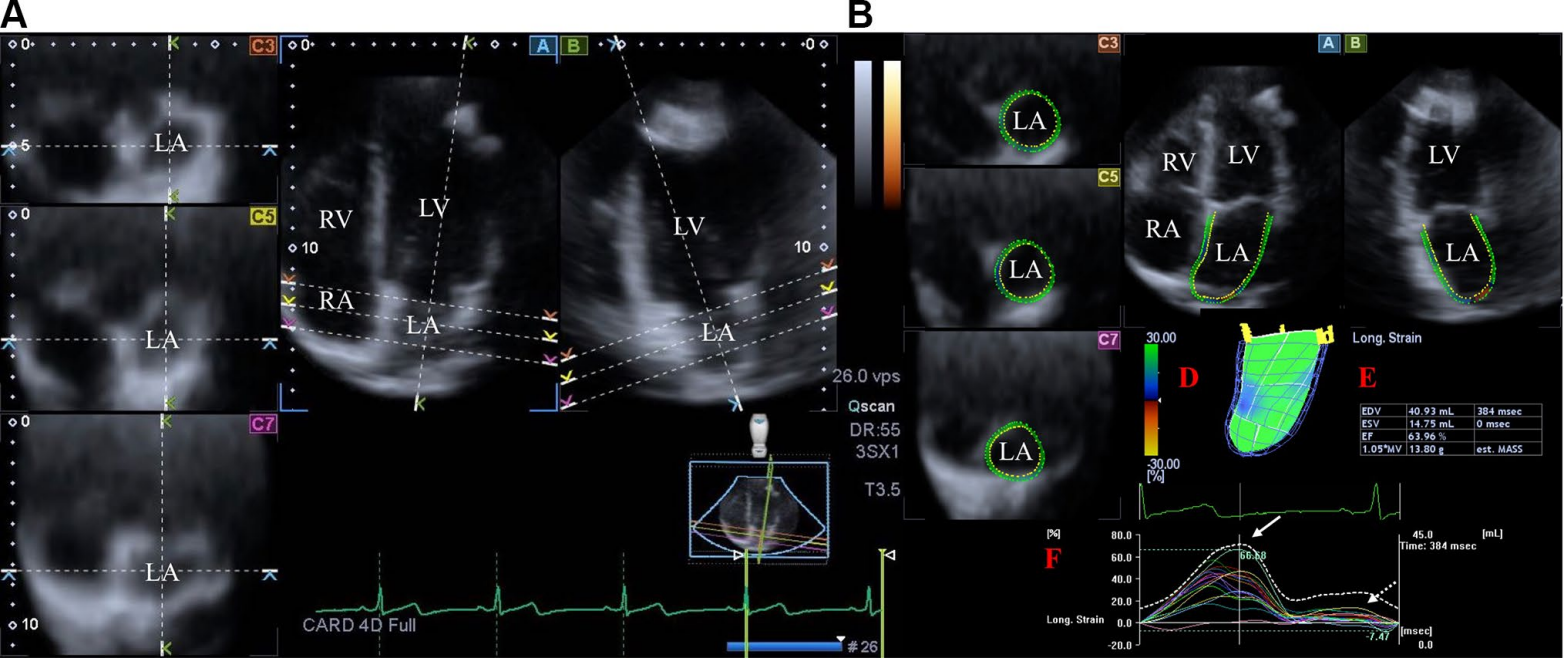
Images from three-dimensional (3D) full-volume dataset showing the left atrium (LA) in a healthy subject are demonstrated (a, b): (*A*) apical four-chamber view, (*B*) apical two-chamber view, (*C3*) short-axis view at basal, (*C5*) mid- and (*C7*) superior left atrial level. On a, dashed lines represent different planes optimalized on the long-axis and cross-section of the LA. On b, the semi-automated LA border definition, 3D “wire” reconstruction of the LA based on 3D speckle tracking echocardiographic analysis (red *D*) and LA volumetric data (red *E*) are presented. Coloured lines represent segmental LA strains while dashed white line represents LA volume changes over the cardiac cycle (red *F*). White arrow represents peak LA strain, while dashed arrow represents LA strain at atrial contraction. *LA* left atrium, *LV* left ventricle, *RA* right atrium, *RV* right ventricle
